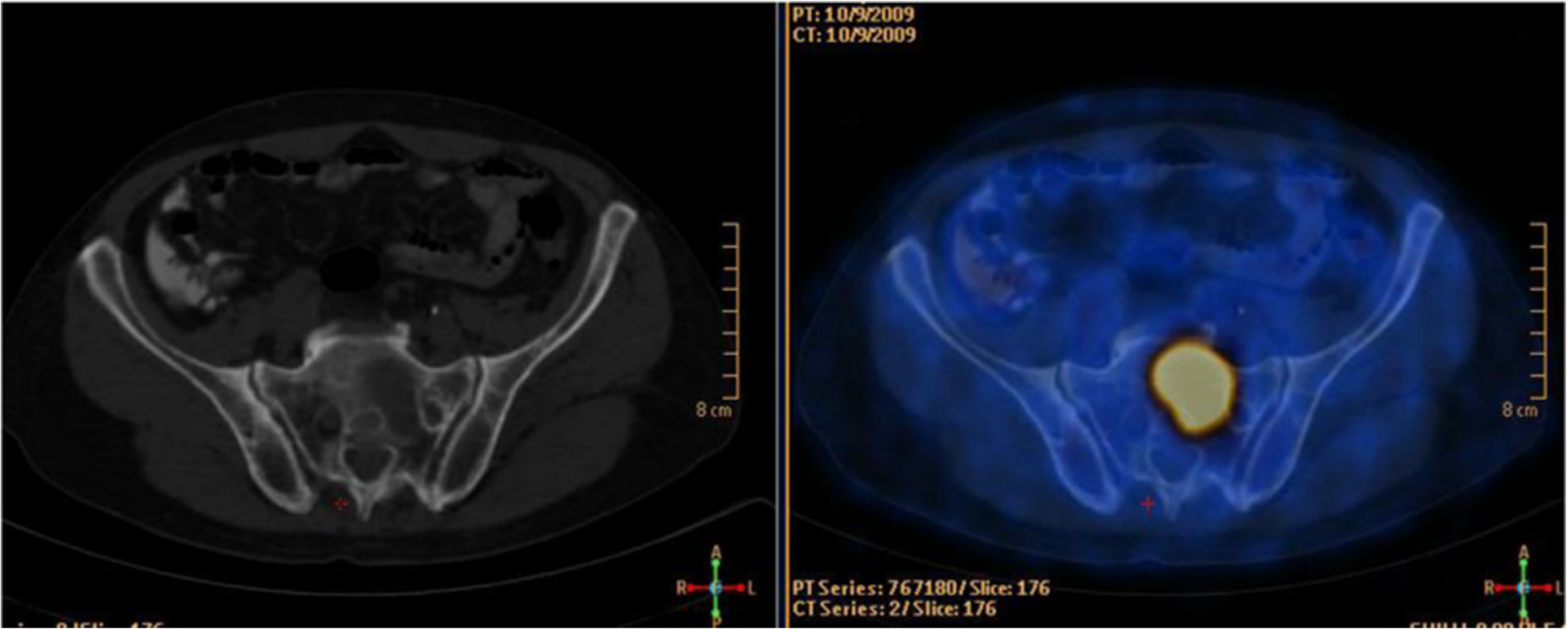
Transaxial CT and fused PET/CT images of a 65-year-old male patient with rectal cancer.^18^F-FDG PET/CT showed intense ^18^F-FDG uptake (SUVmax: 11.3) in sacral metastases, which could be easily missed by ceCT.
